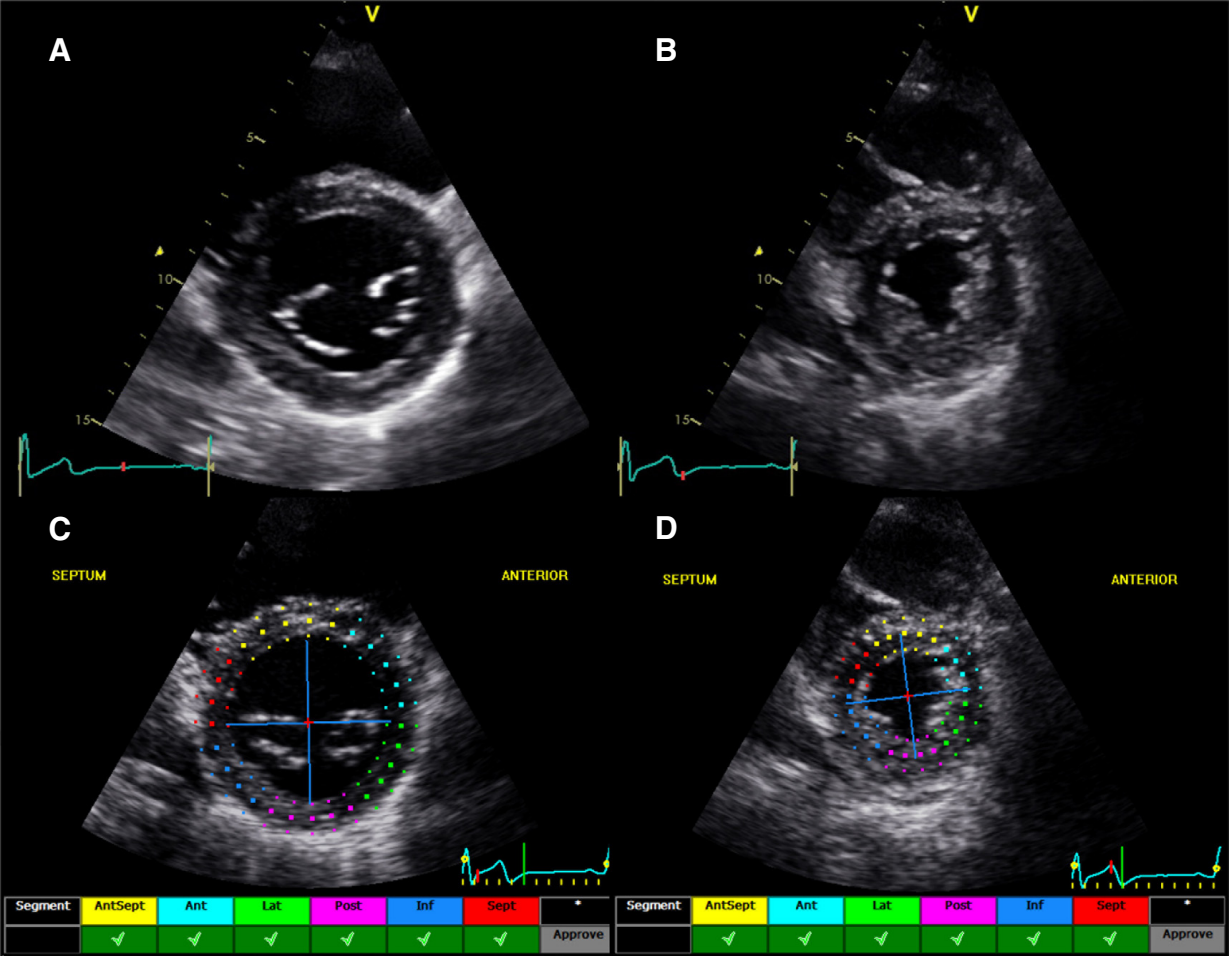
Representative short axis images and speckle tracking imaging. Strict imaging criteria was utilized in order to standardize all parasternal short axis images. (A) An adequate basal image was defined by the presence of full thickness myocardium surrounding the mitral valve at end systole. (B) The left ventricular (LV) apex was obtained by moving the transducer one to two intercostal spaces caudally from the basal position to align with the apical short axis with no visible papillary muscles that closely approximated an end diastolic ratio of LV cavity diameter to total LV diameter of 0.5, as described previously (Weiner et al. 2010). The endocardium was traced manually on the two‐dimensional image and the speckle tracking software automatically tracked myocardial motion and only acceptable tracking was accepted as shown in (C) basal and (D) apical short axis images.
